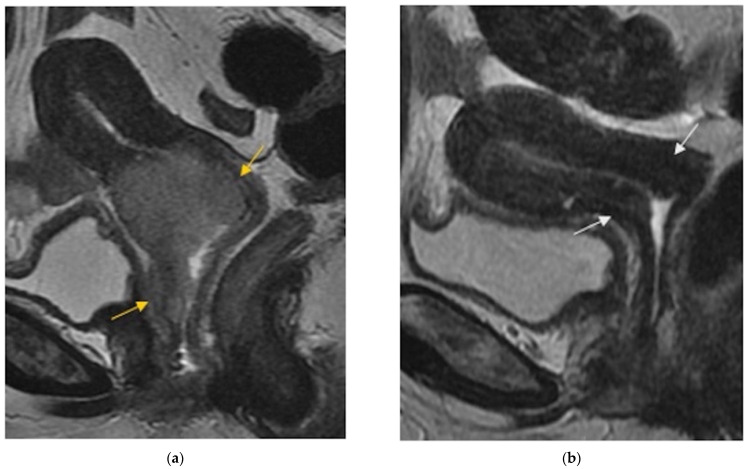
(a) Sagittal FSE T2-weighted image shows soft tissue with high signal intensity consistent with a cervical tumor extended to the vagina (arrows). (b) Sagittal FSE T2-weighted image shows reconstitution of the normal T2-weighted hypointense cervical stroma (arrows), with disappearance of the tumoral mass after chemoradiation therapy.
